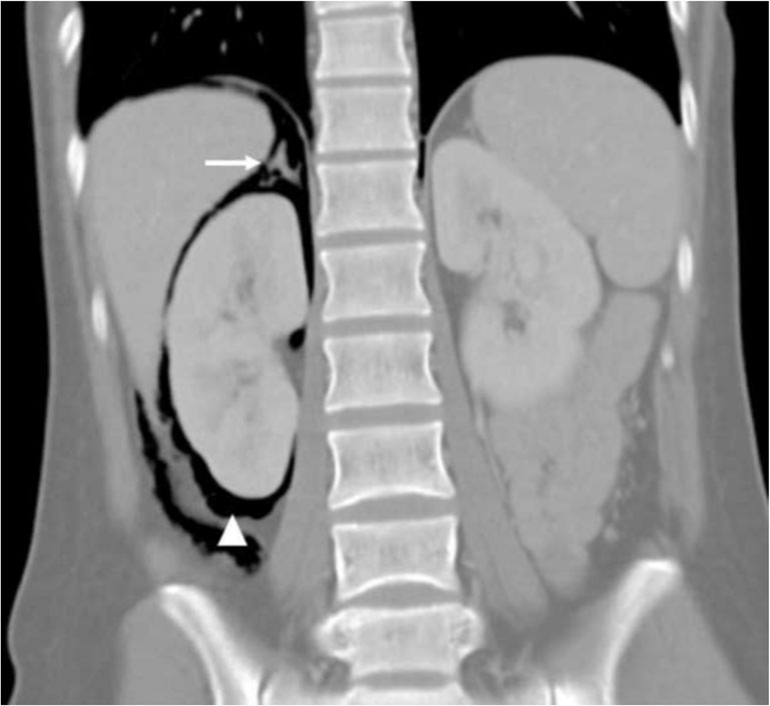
Conal portal venous CT shows gas in the perinephric space (*arrowhead*), demonstrating its longitudinal conal orientation and that it contains both the kidney and the adrenal (*arrow*). The gas was secondary to a perforation during colonoscopy
